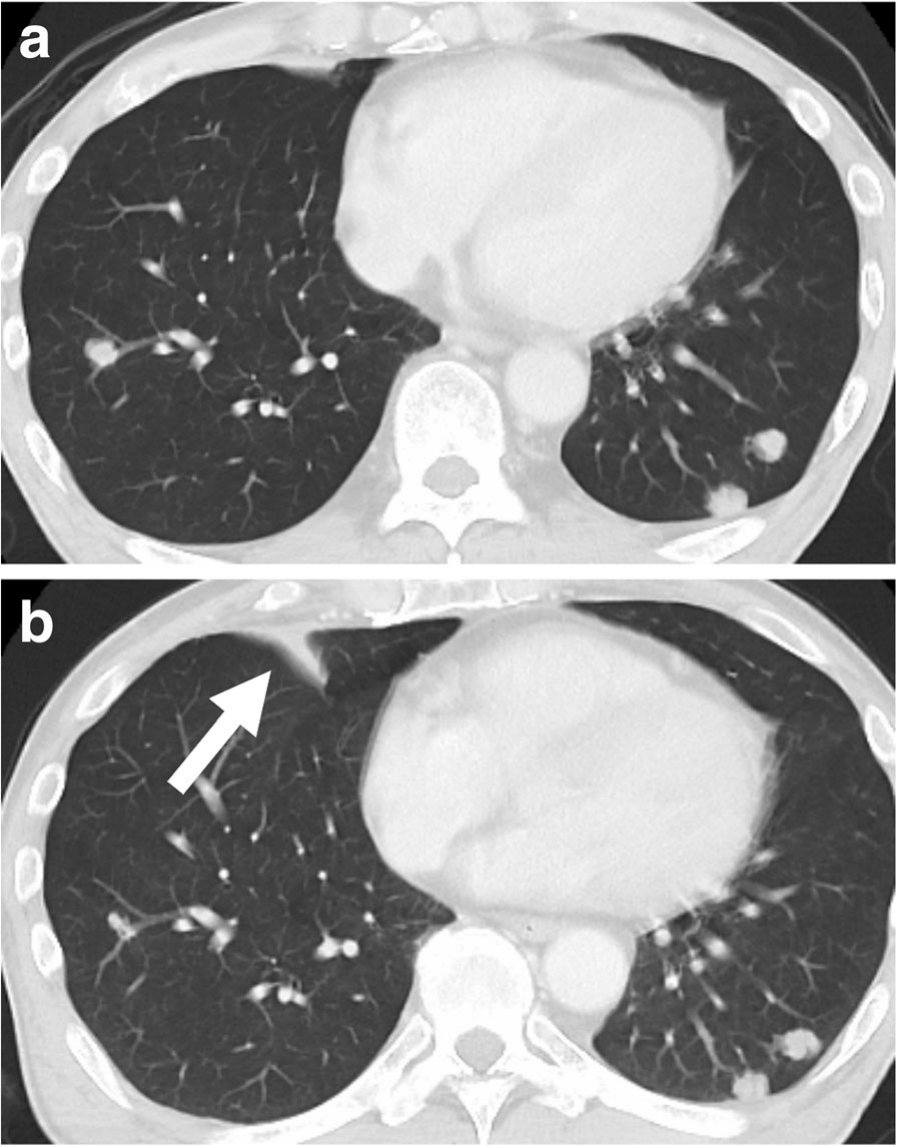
Chronological changes in the lungs for Case 1 as shown usingcontrast-enhanced computed tomography. a Multiple pulmonarymetastases in both lungs. b Consolidation suggesting inflammationapart from pulmonary metastasis. The arrow is pointing to the part of the tuberculosis infection
